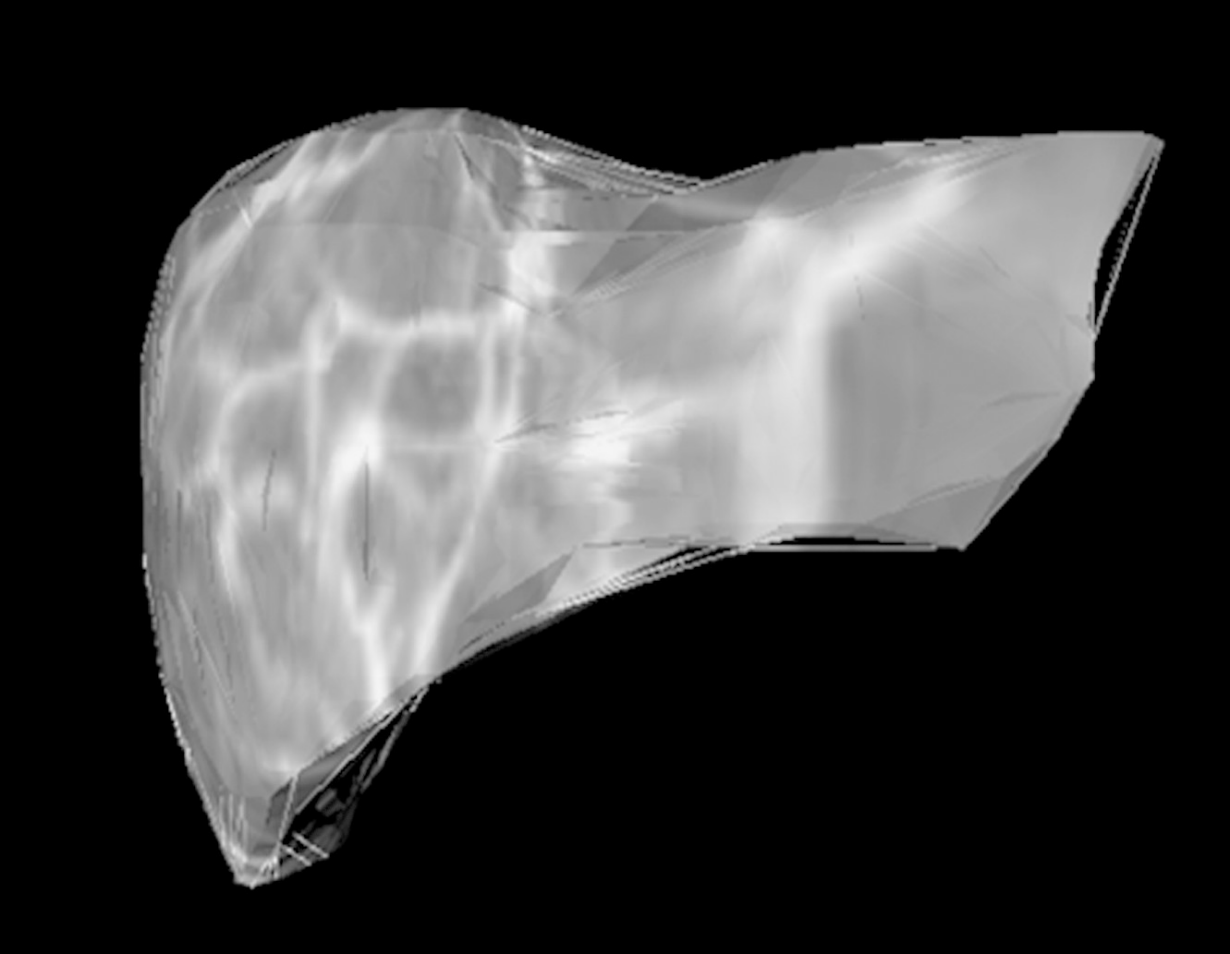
3D image acquired by magnetic resonance imaging.
